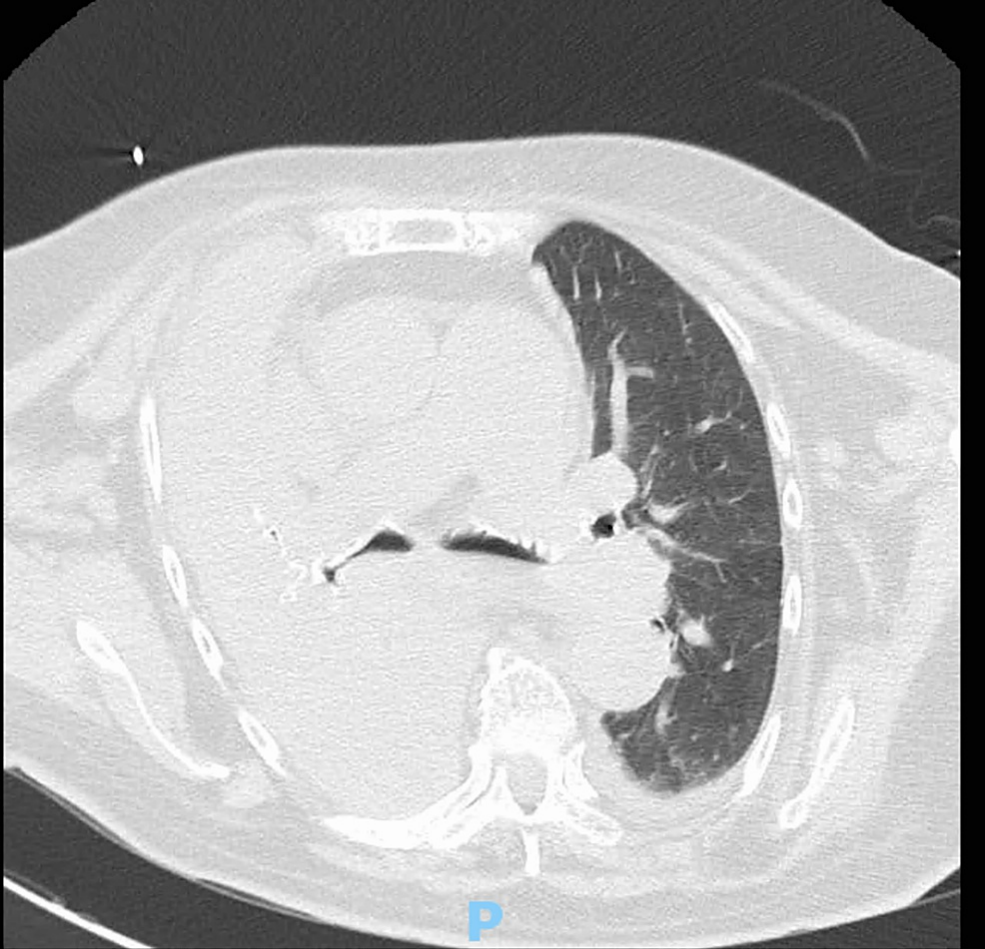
A CT with angiography of the chest showing significant right pleural effusion with associated right-lung collapse.
